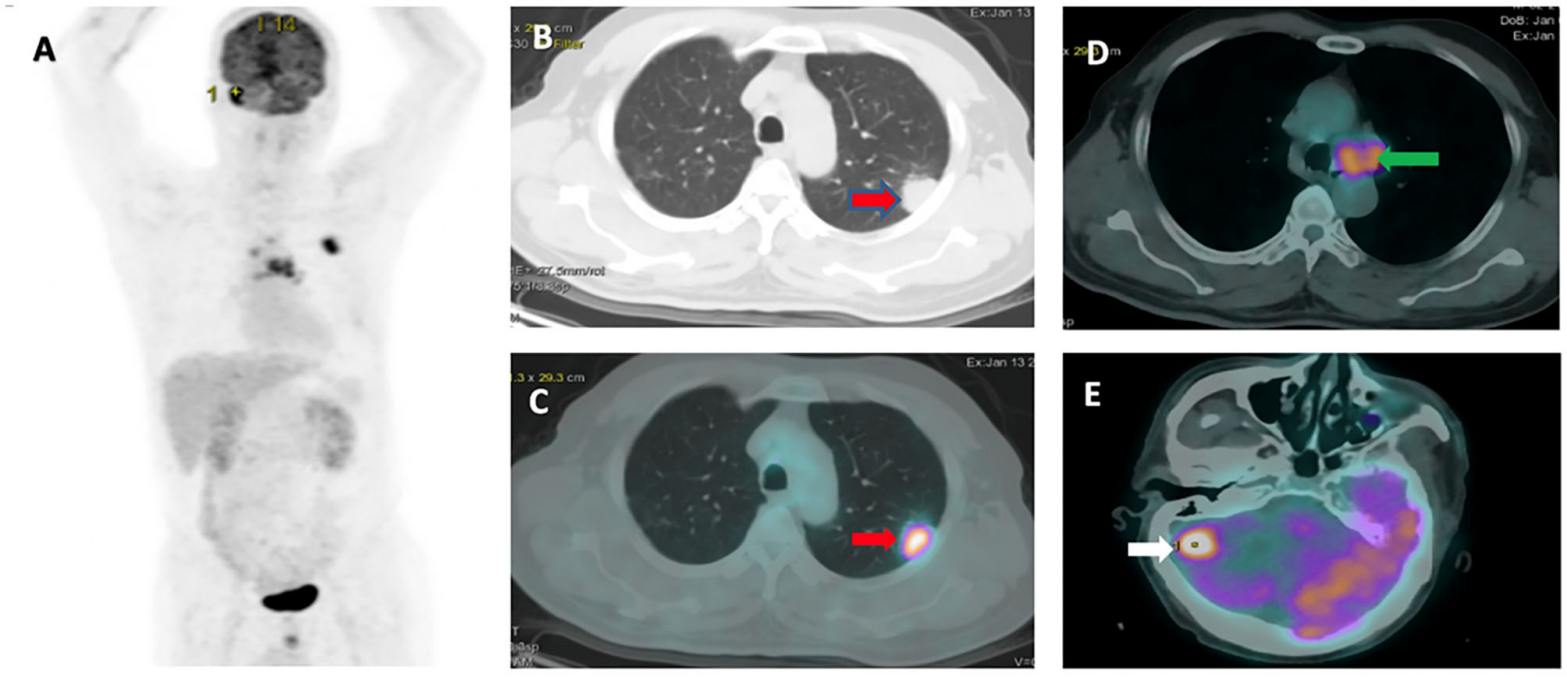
Representative ^18^F-FDG PET/CT images: (A) PET image. (B,C) Pleural tumor invasion was detected in the upper left lung with SUV_max_ or 10.17 (red arrow). (D) Mediastinal node metastasis (green arrow). (E) Brain metastasis (white arrow).
